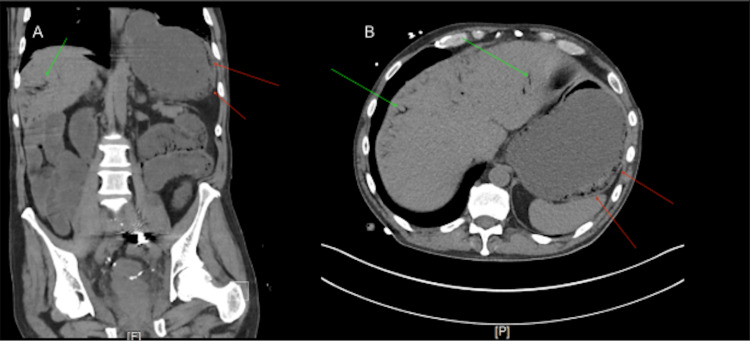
The CT scan demonstrating the coronal (A) and axial (B) views. Red arrows: Air within the stomach wall secondary to gas-producing bacteria. Green arrows: Portal venous gas translocation of air from the stomach wall into portal hepatic circulation.
